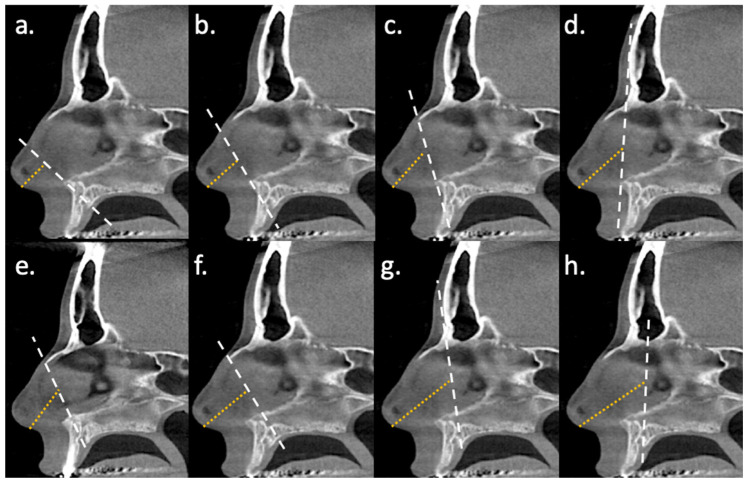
Identification of eight planes perpendicular to the curved airflow in the nose in a mid-sagittal section. The white dashed line indicates the selected oblique plane using as pivot point the anterior nasal spine in (a–d), the most cranial part of the premaxilla at the level of the anterior borders of the ascending processes of the maxilla in (e), and the anterior edge of the superior ostium of the incisive canal in (f–h). The yellow dashed line indicates the distance from the midline of the caudal border of the columella to the midline of the CT-CA mid-sagittal design. The corresponding cross-sectional areas of the nasal airways are as follows: (a) CT-CSA_ant-30_, (b) CT-CSA_COT-2_, (c) CT-CSA_ant-60_, (d) CT-CSA_ant-90_, (e) CT-CSA_COT-3_, (f) CT-CSA_post-50_, (g) CT-CSA_post-80_, and (h) CT-CSA_post-100_.
